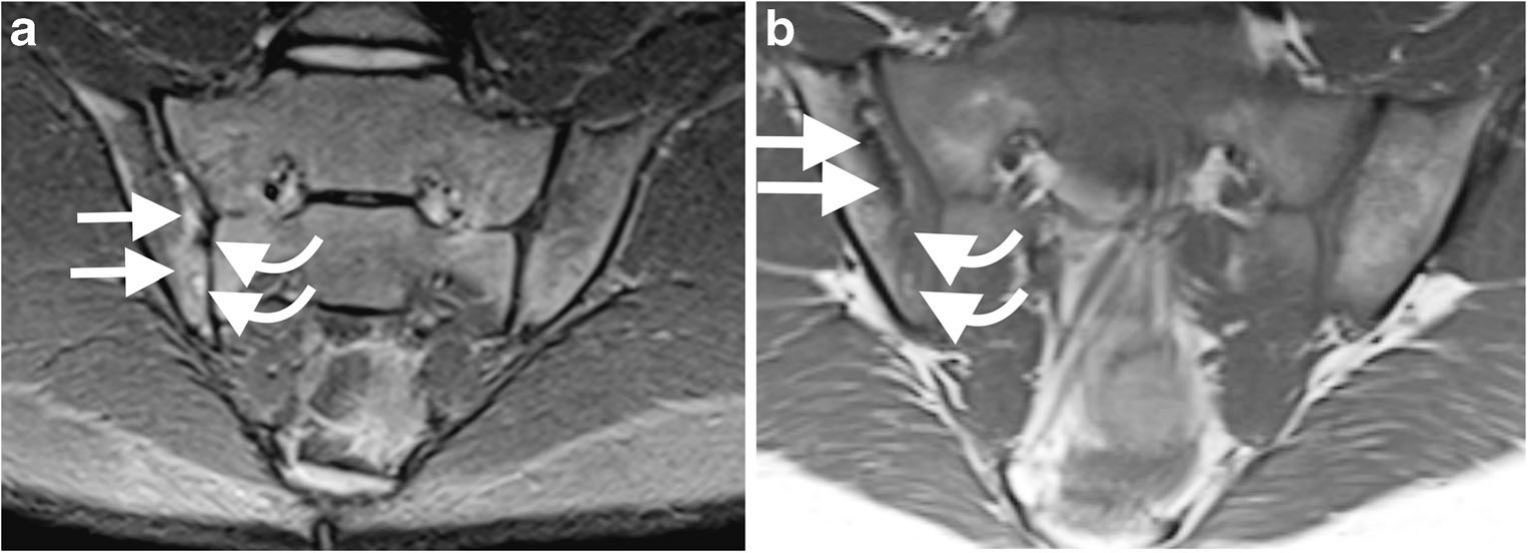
A 17-year-old boy with juvenile spondyloarthritis. a-b Coronal oblique short tau inversion recovery (STIR) spin echo (TR 4,000 ms, TE 36 ms) (a) and T1-weighted spin echo (TR 679 ms, TE 12 ms) (b) images at slightly different locations in the joint demonstrate multiple erosions on the iliac side of the right sacroiliac joint (*curved arrows*) with surrounding oedema (*straight arrows*), which is high signal on (a) and low signal on (b)
